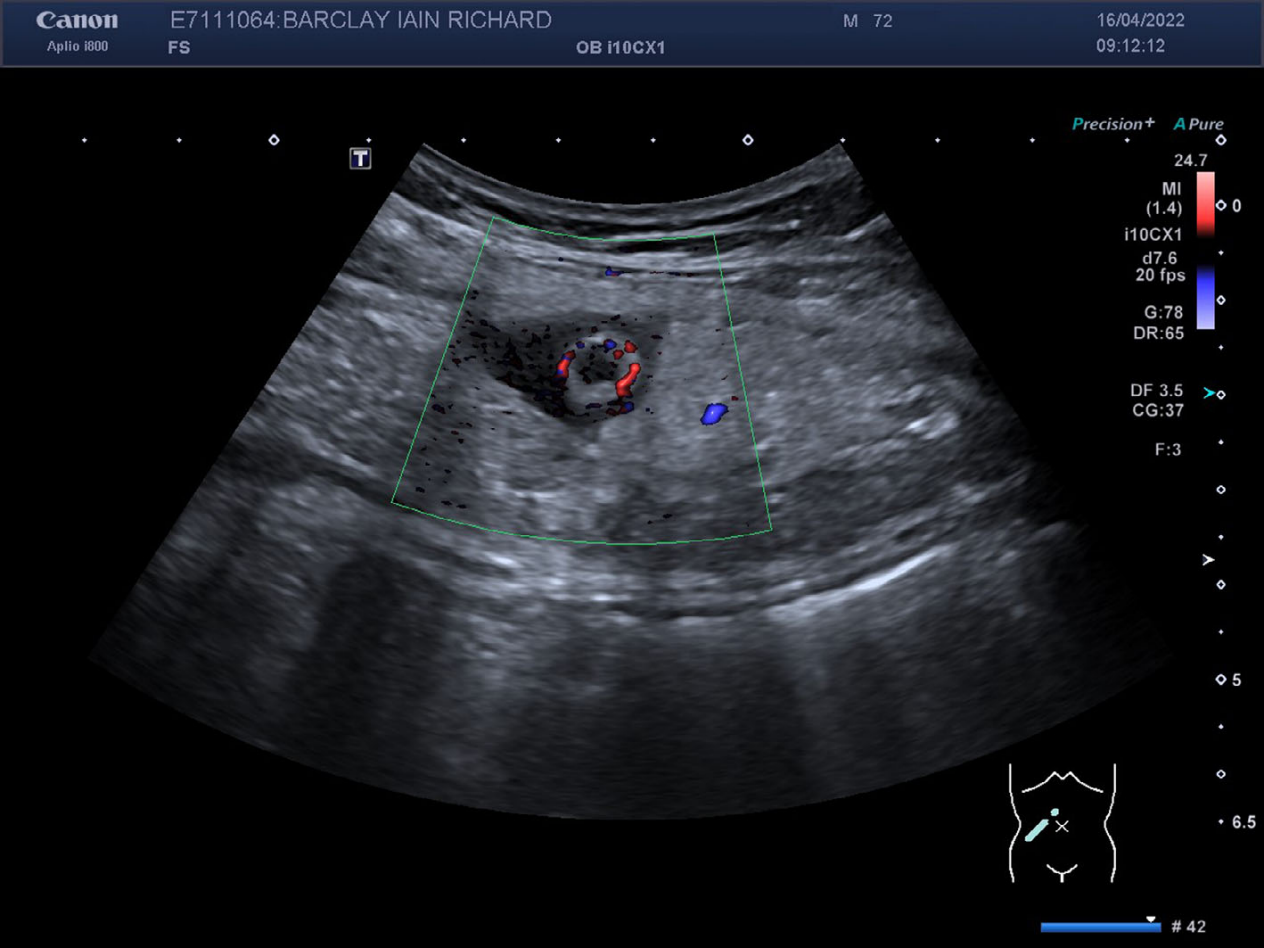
An abdominal ultrasound confirming appendicitis within a spigelian hernia.
